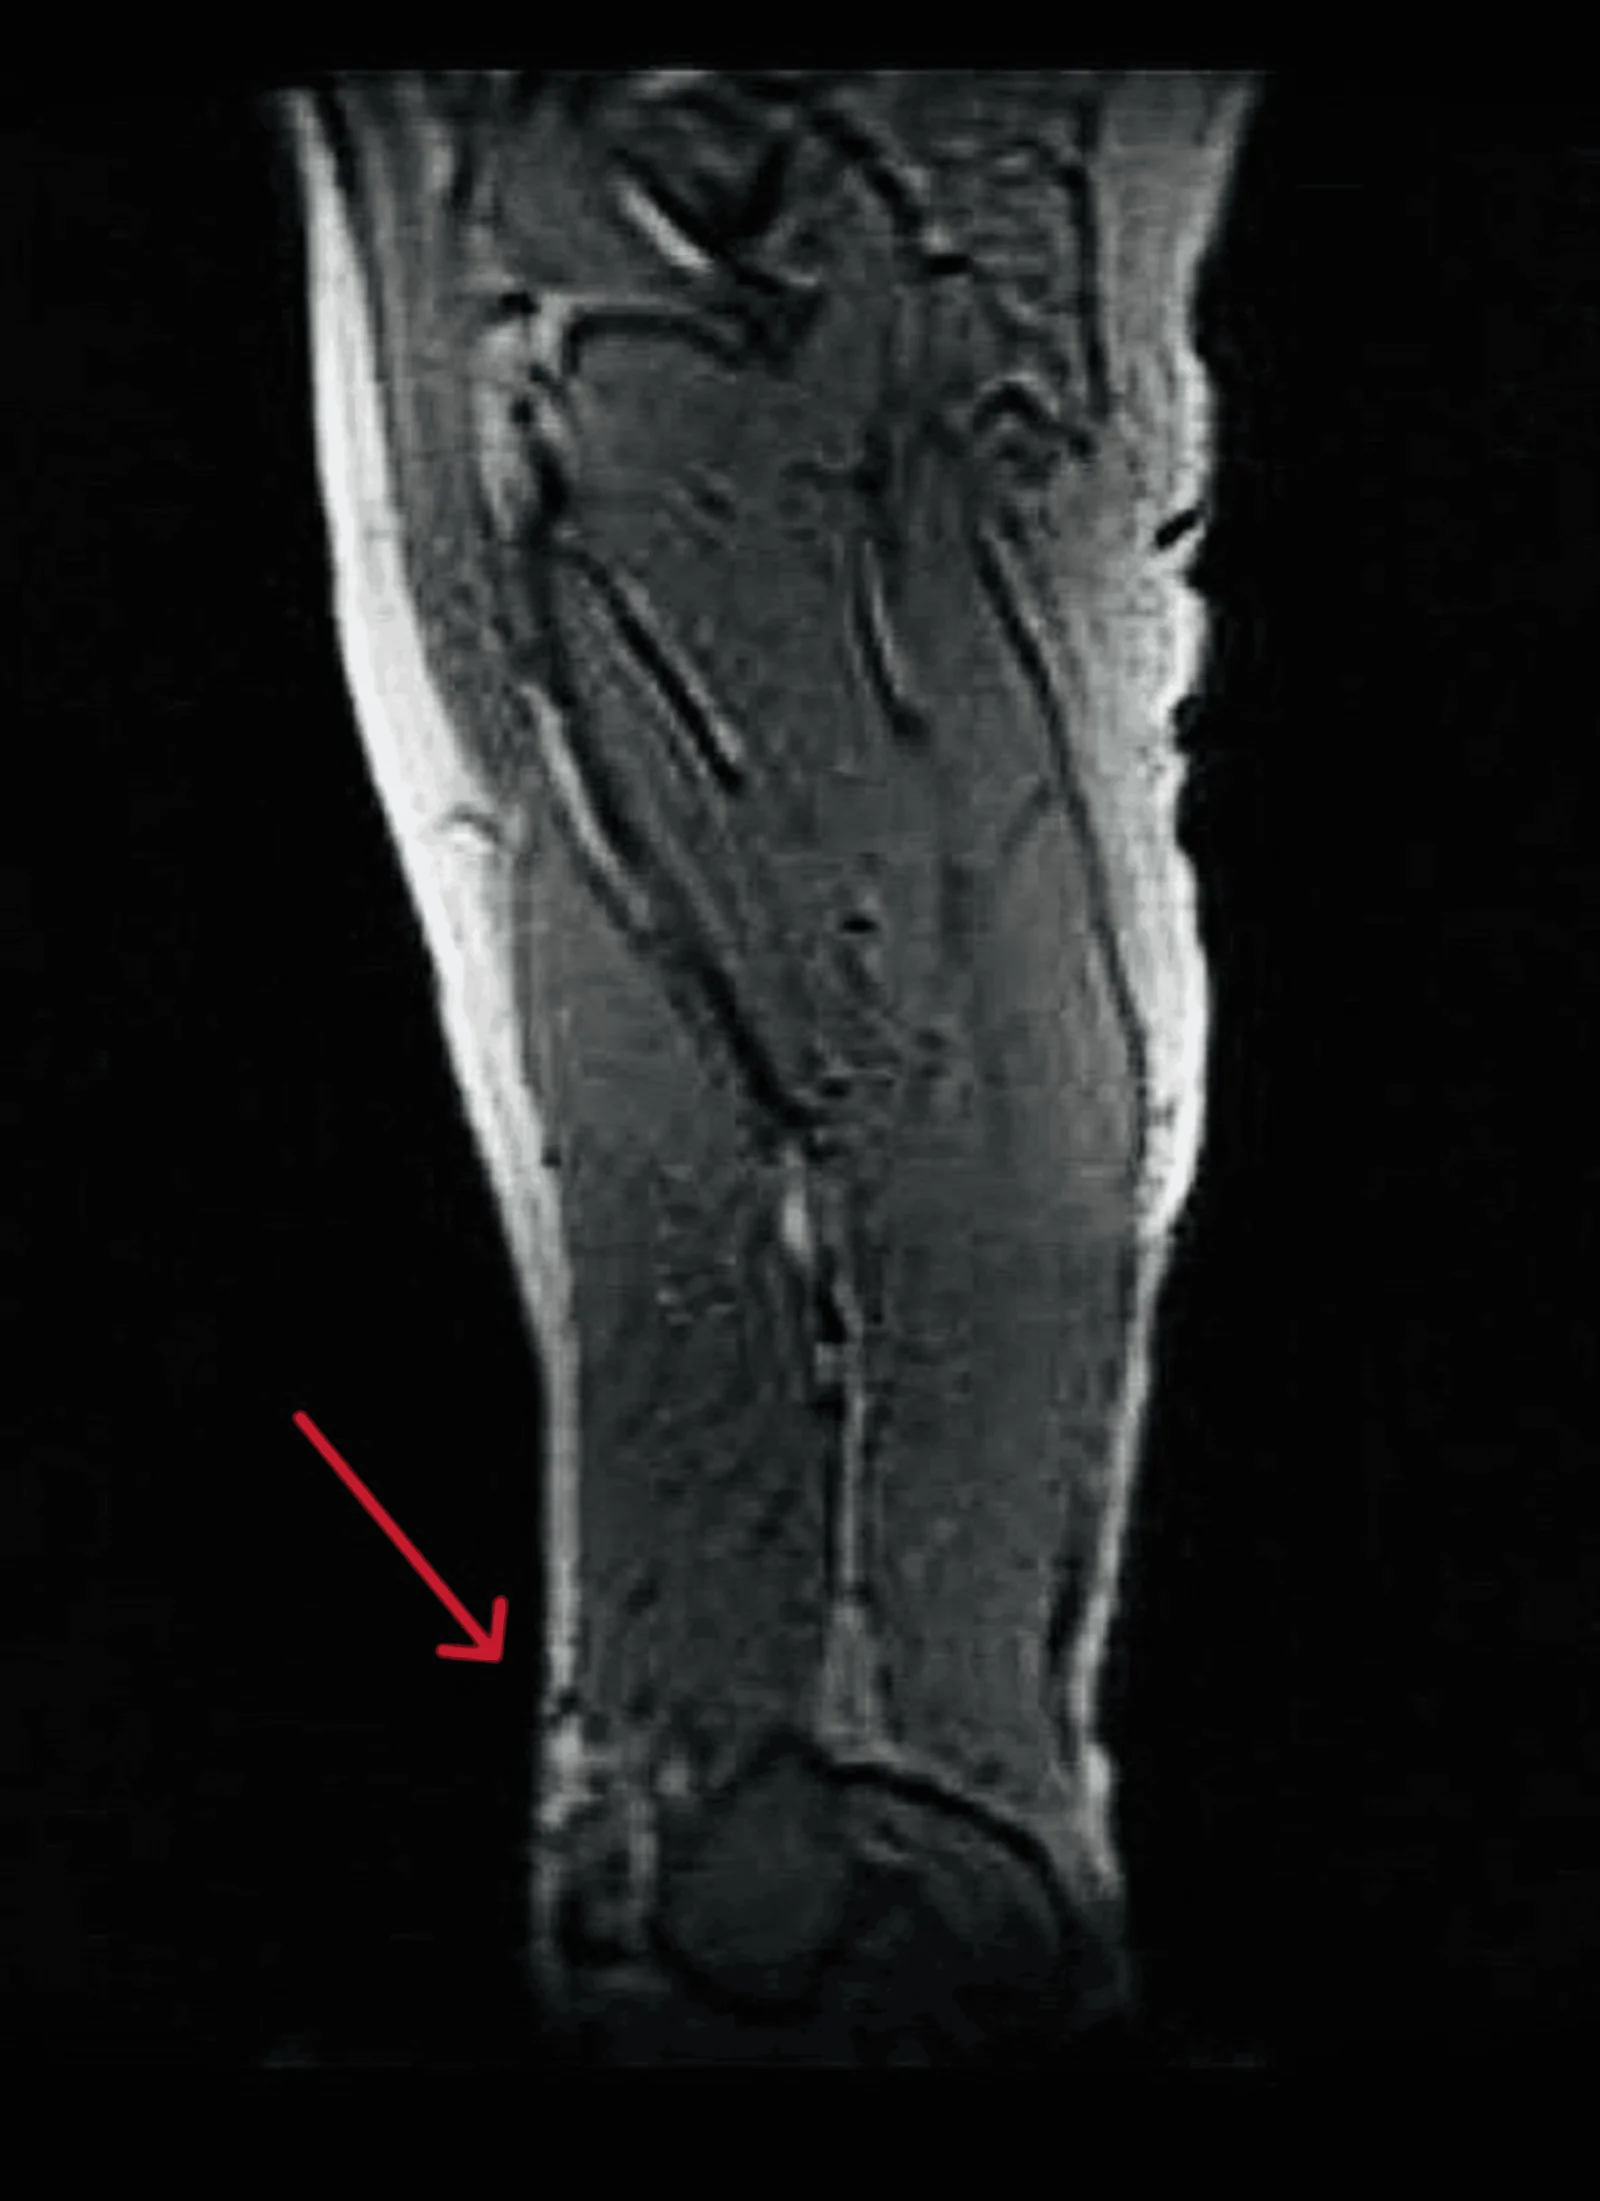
Sagittal T2-weighted MRI of the left knee demonstrating discontinuity of the quadriceps tendon at its insertion on the superior pole of the patella (arrow).
